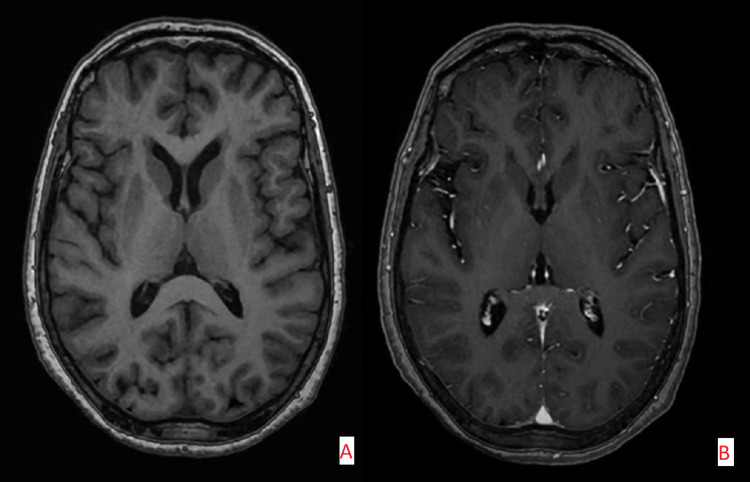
Pre- and post-contrast T1-weighted sequences Pre-contrast T1-weighted image (A) and post-gadolinium T1-weighted image (B) showing no mass-like lesion, no significant mass effect, and no abnormal enhancement.
